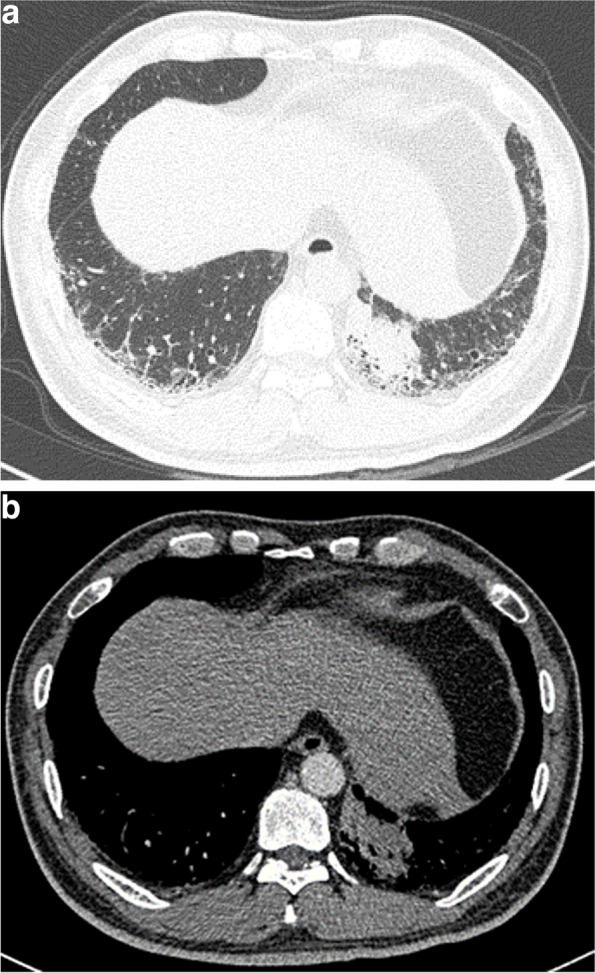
A 54-year-old male smoker with stage IV adenocarcinoma located in the left lower lobe. Pre-treatment chest CT (a) demonstrated ground-glass opacity, reticulation, traction bronchiectasis, and honeycombing predominantly located in bilateral lower lobes, in accordance with subpleural fibrotic ILA. The tumor was located in ILAs and presented the lobulation sign (a, b). The patient underwent fine needle aspiration biopsy, with a pathological diagnosis of adenocarcinoma without EGFR mutation. He was died 300 days after the diagnosis of lung cancer. CT, computed tomography
